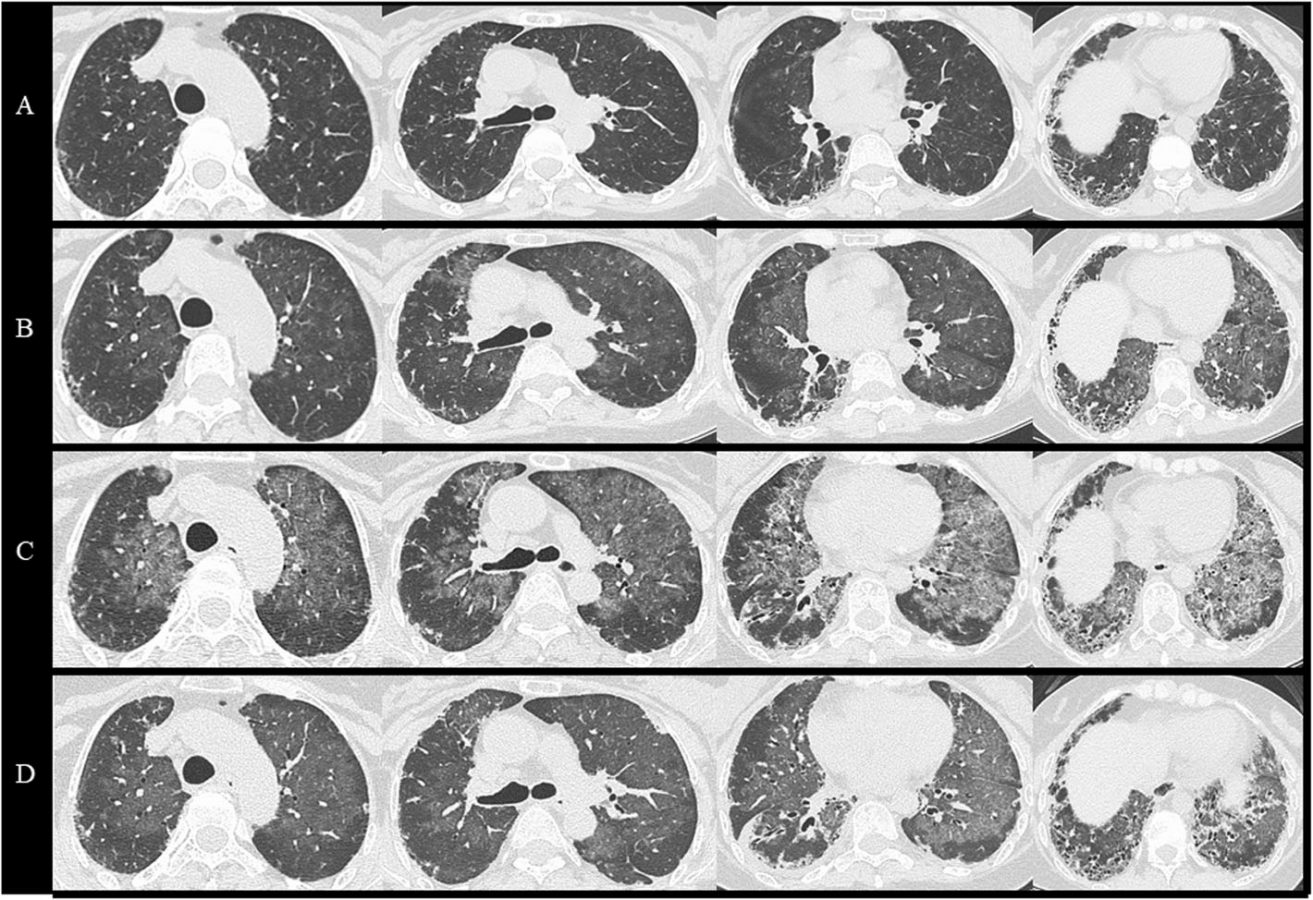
Chest CT findings. A Four months before admission, her chest CT showed a subpleural and basal predominant reticular pattern with peripheral traction bronchiectasis. B On admission, extensive GGOs with geographic distribution appeared bilaterally. C Immediately before WLL, GGOs worsened further in both lung fields four months after the diagnosis. D After WLL, GGOs improved significantly with no change in the underlying interstitial pneumonia.
